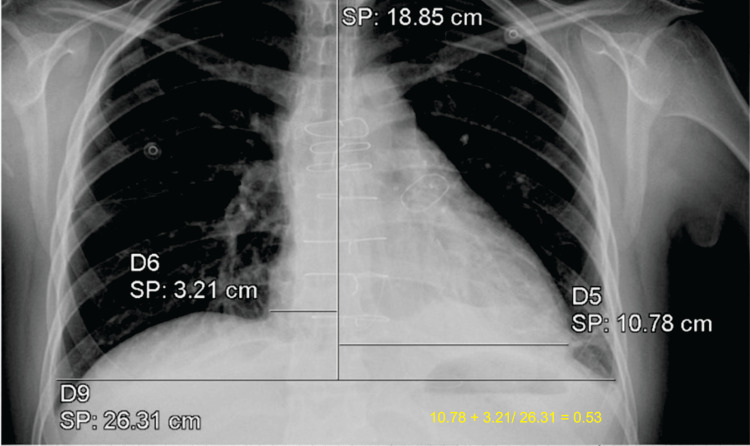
Chest radiography Chest radiography showed cardiomegaly stage 1 with a cardiothoracic index of 0.53.
